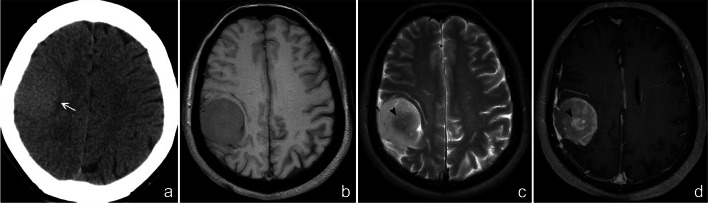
Imaging features of the “yin-yang sign” in MA. a–d CT scan and MRI images of MA at the convexity of the right parietal brain. a head CT scan of tumor parenchyma showed iso-intensity with no calcification in the lesion (arrow); b signal of tumor parenchyma in T1WI showed iso-signal (using cerebral cortex signal as the reference); c, d T2WI and T1WI enhancement showed that areas within the tumor having low signals in the T2WI were more significantly enhanced in the T1WI (black arrow), also known as the “yin-yang sign”
